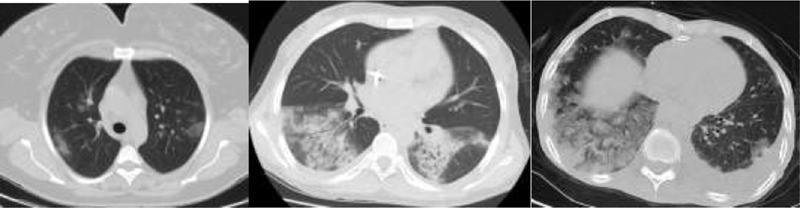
Stages of Covid-19 infection according to computed tomography findings (
